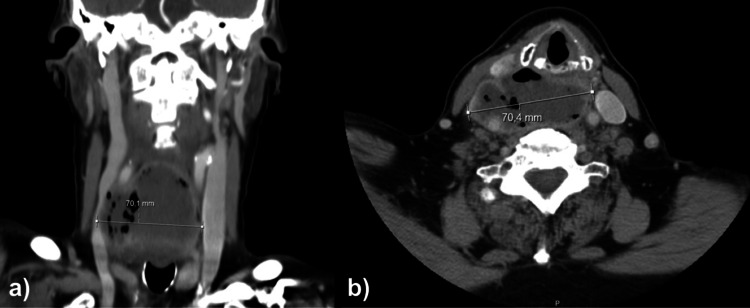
a) Image of the abscess on cervical computed tomography in coronal section. b) Image of the abscess on cervical computed tomography in cross-sectional.
